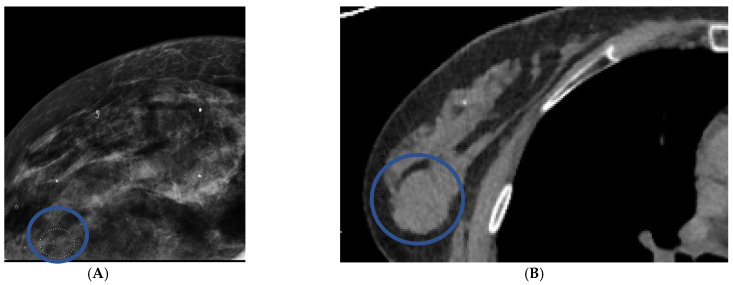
Right upper outer quadrant microcalcifications on craniocaudal mammography were recommended for biopsy (A). Axial chest CT performed 128 days prior to the mammogram recommended biopsy for a 20 mm right upper outer quadrant mass (B). Biopsy results at the time of mammography were ductal carcinoma in situ.
